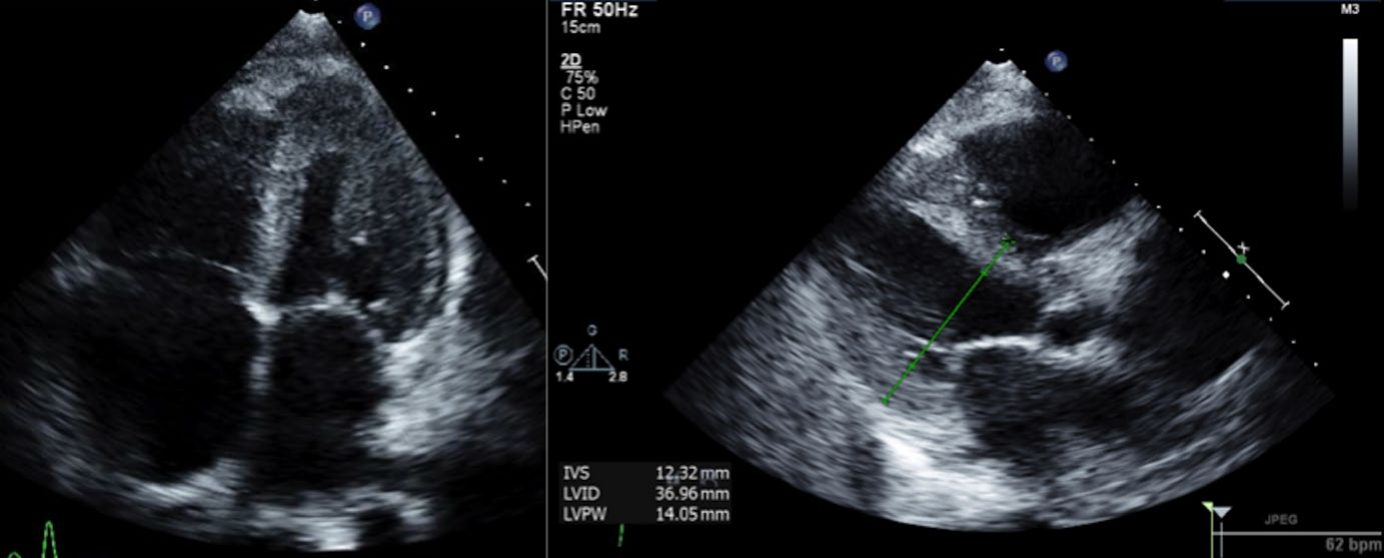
Apical 4 chamber and parasternal long axis echocardiographic views showing left ventricular hypertrophy (posterior and septal wall thickness—14 and 12 mm, respectively) along with severely increased right ventricular and right atrial cavity size
